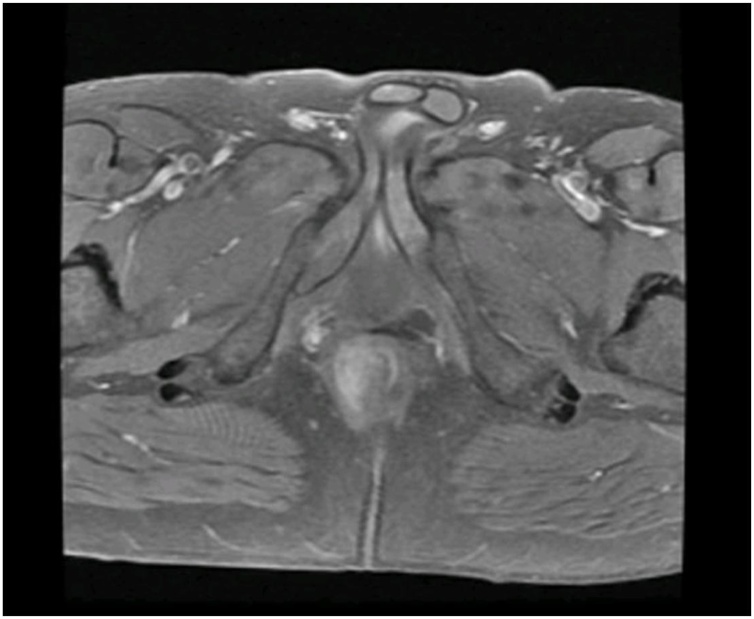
Transverse plane of pelvic MRI depicting the perianal mass.
